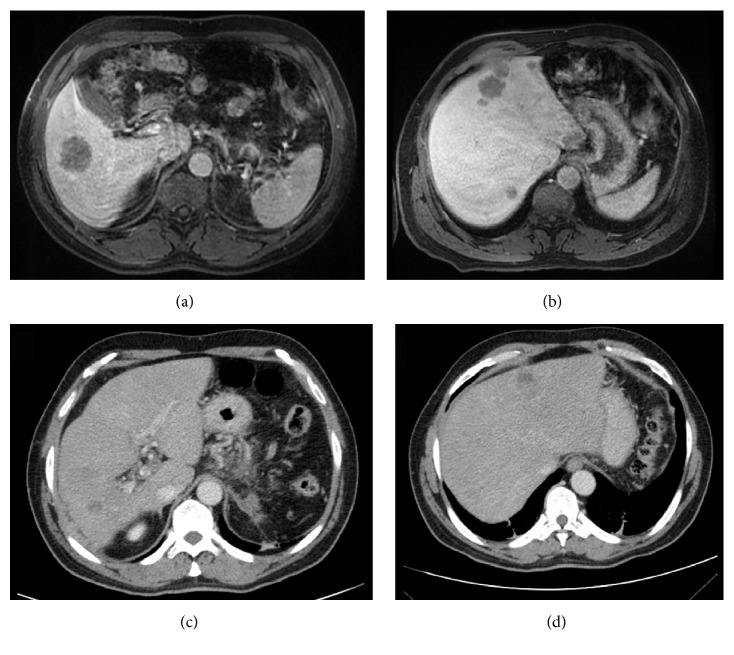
Comparison of baseline magnetic resonance imaging (MRI) (August 1, 2015) with computed tomography (CT) imaging performed on December 16, 2016. (a, b) Axial MRI images, portal phase, showing the hepatic nodules in the right (a) and left (b) lobes at admission. (c, d) Axial CT images, portal phase, showing regression of the hepatic nodules in the right (c) and left (d) lobes 15 months after the second SIRT and after several lines of chemotherapy.
